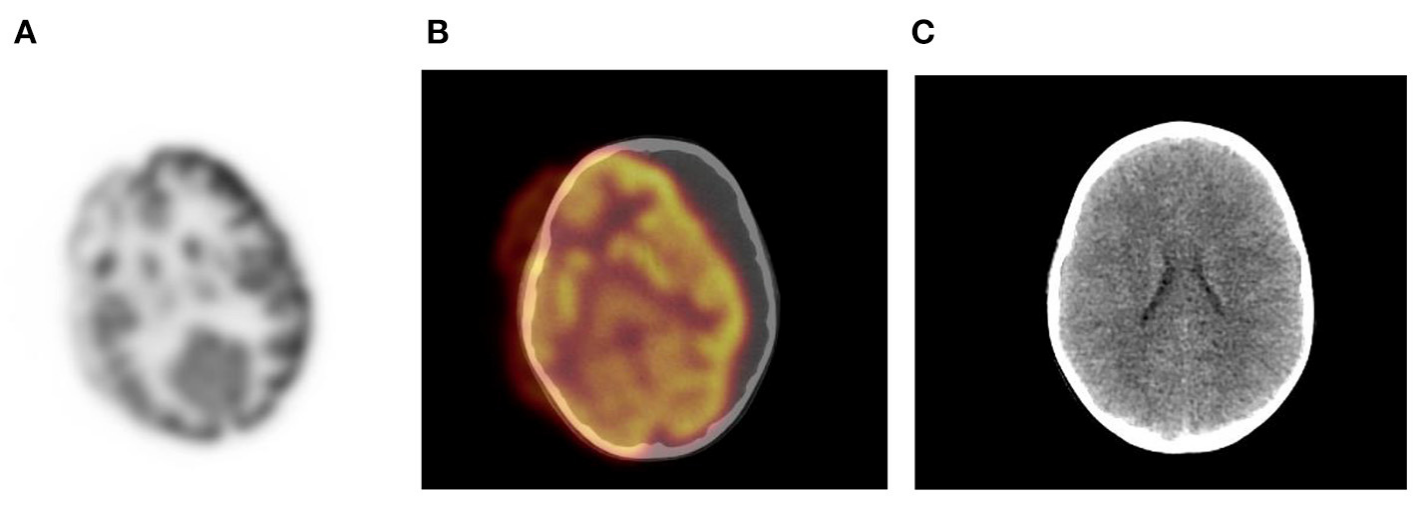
Motion artifact of the brain on axial PET and PET/CT images (A,B). No obvious pathology is seen on the non- contrast axial CT component (C) of the PET study.
